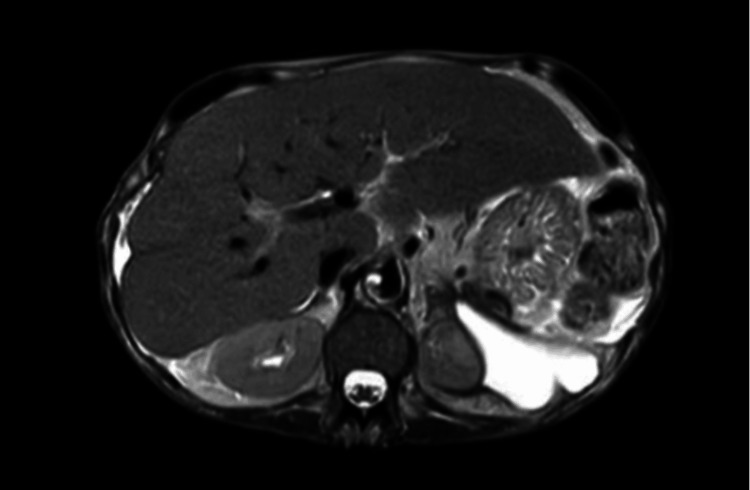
T2-weighted MRI showing a chronic pseudocyst in the left retroperitoneal space, causing mass effect upon the superior pole of the left kidney
